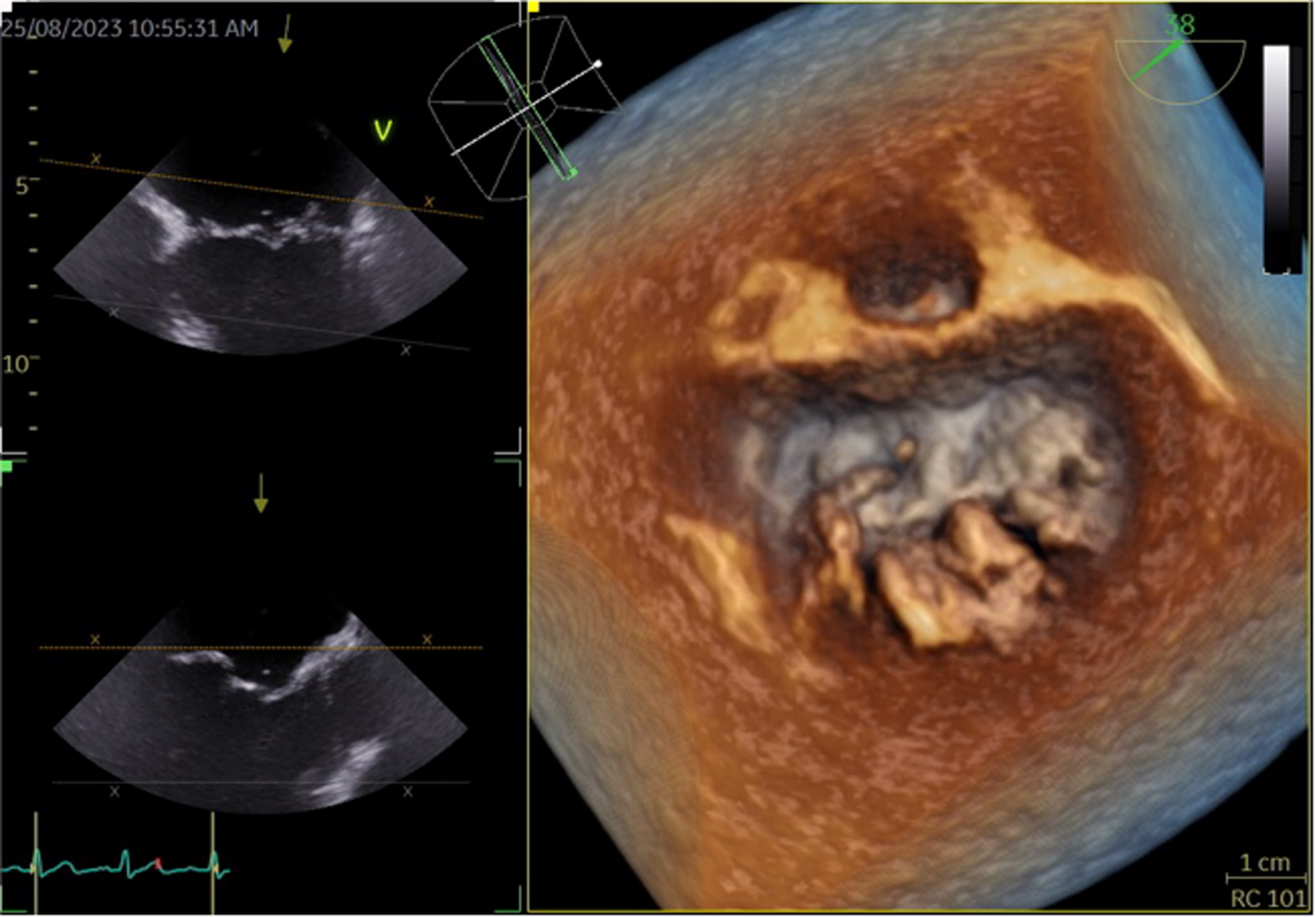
Mitral valve reconstruction from a three‐dimensional (3D) transesophageal echocardiography suggestive of vegetation in the posterior leaflet.
